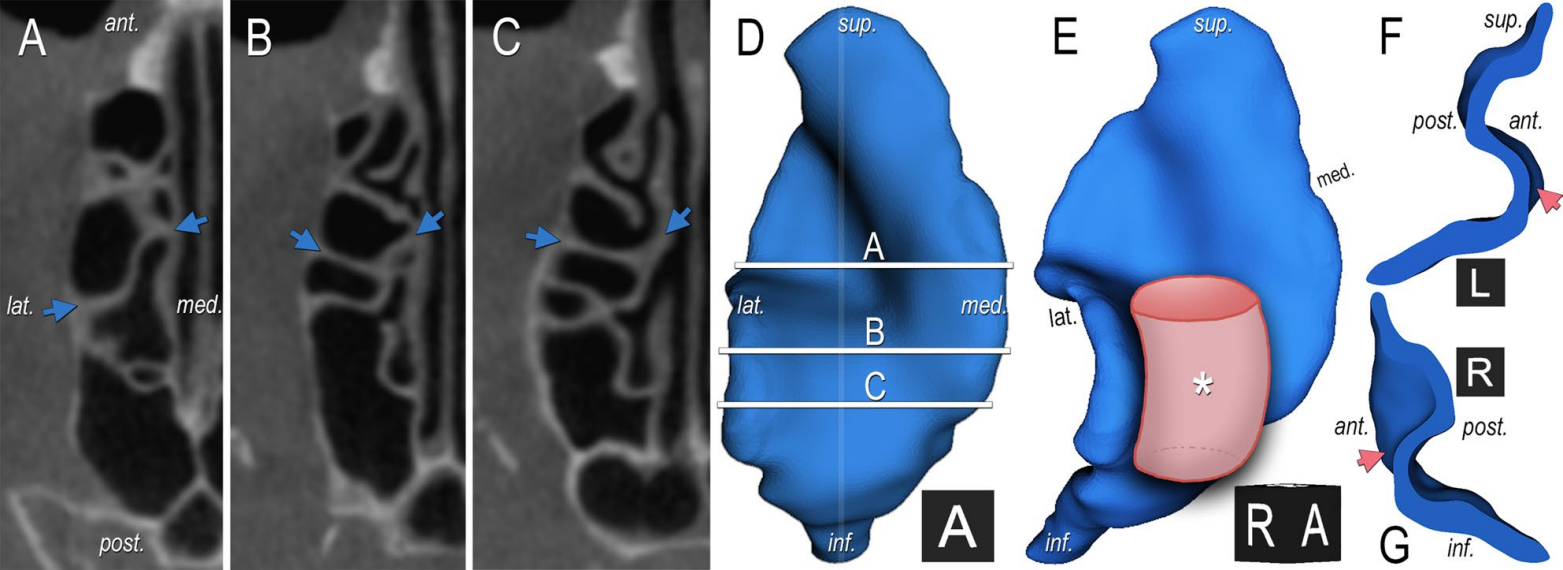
Generation of the concave/convex segment. The right ethmoid complex on axial CBCT reconstruction with a sigma (A) and a concave/convex segment (B–C) of the 3BL enclosed by *blue arrows* (see (A) for orientation of A–C. D Depicts the anterior view of the 3BL’s 3D shape (identical to Fig. 5A); *horizontal lines* match to slices of A–C. E Depicts the circa 45° anterolaterally rotated view of the 3BL’s 3D shape (identical to Fig. 5B). The 3BL of D was split along the *vertical line* to a lateral (F) and medial (G) part. Black cubes—*A* anterior, *L* left, *R* right. The facilitating factors of the concave/convex segment can be identified (B,C): the posterior ethmoid is wider than the anterior; the attachment of the 3BL to the orbital lamina of the ethmoid bone is approximately 90°; the medial 3BL border is slightly anterior compared to the lateral border and the middle part is posterior; a posterior ethmoidal cell produces laterally an anterior shift of the 3BL. Consequently, from an anterior point of view the concave/convex segment seems to be shaped by an object like a “bent tube” (E); impact demonstration: *). The cut edge is a convex curvature (F–G). On axial images, the shape is concave with anterior protrusions on the sides ((B–C,F–G) with *pink arrows*).
